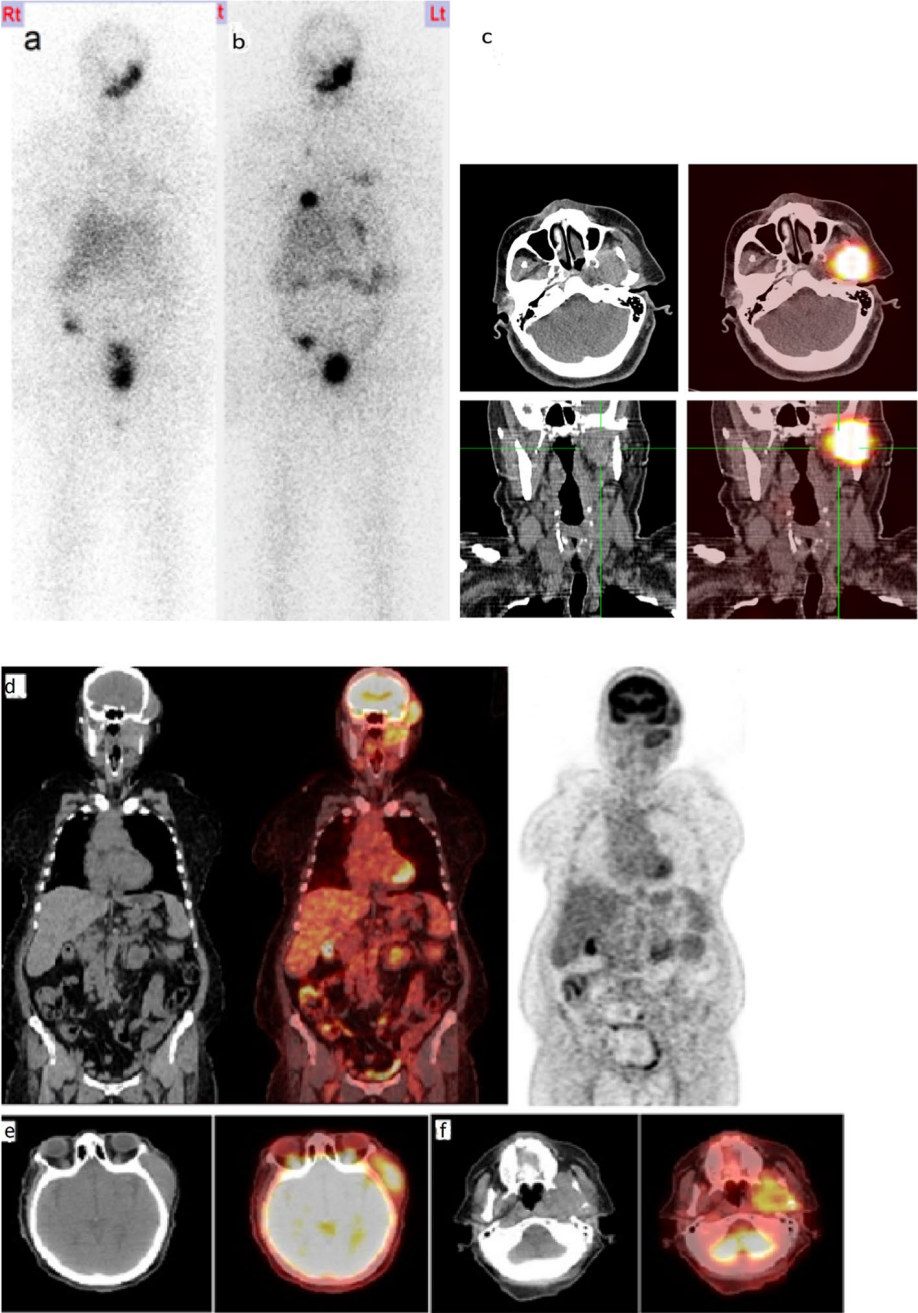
a Post-therapy WBI scan showed uptake in left submandibular area misinterpreted as salivary gland activity, b follow-up post-therapy WBI scan revealed disease progression with increased uptake in submandibular region and increase in number and intensity of pulmonary lesions, c SPECT/CT images show iodine avid pterygoid muscles metastasis. d Whole body^18^F FDG-PET/CT coronal images show intense FDG uptake by left temporalis and pterygoid muscles indicating muscle metastases, e trans-axial images show increased FDG uptake by left temporalis muscle and f trans-axial images show increased FDG uptake by left pterygoid muscles
